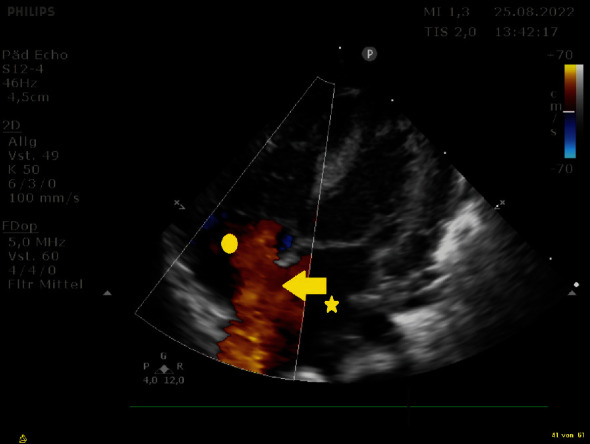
Echocardiography after BAS: a large ASD with left-to-right-shunting is present (arrow); the left atrium is decompressed (star) and the right atrium is larger (dot).
